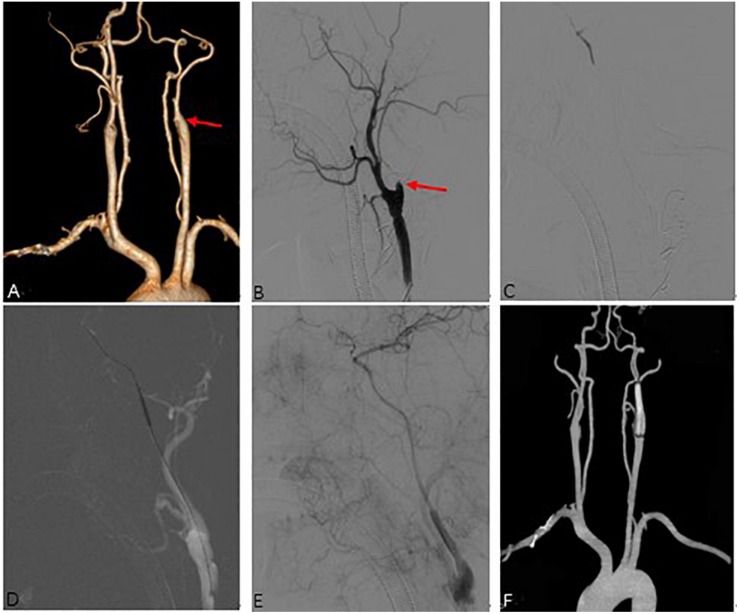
Representative Case 3. A 53-year-old man with right-sided limb weakness underwent hybrid surgery for internal carotid artery occlusion (ICAO). (A) Preoperative computed tomography angiography (CTA) showed left total ICAO (red arrow). Intraoperative angiography showed (B) an artificial stump (red arrow) after carotid endarterectomy and distal true lumen of ICA (C). Successful recanalization (E) was achieved after balloon expansion (D) and deployment of the stent. (F) CTA showed a patent ICA at 3 months after the surgery.
